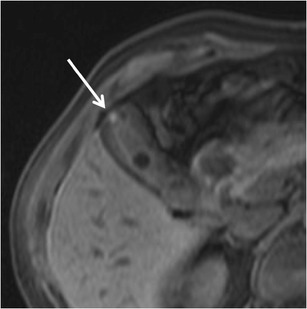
MRI of gallbladder adenomyomatosis: T1-hyperintense Rokitansky–Aschoff sinuses. Rokitansky–Aschoff sinuses (*arrow*) may appear hyperintense on T1-weighted images if containing concentrated bile or calcifications
